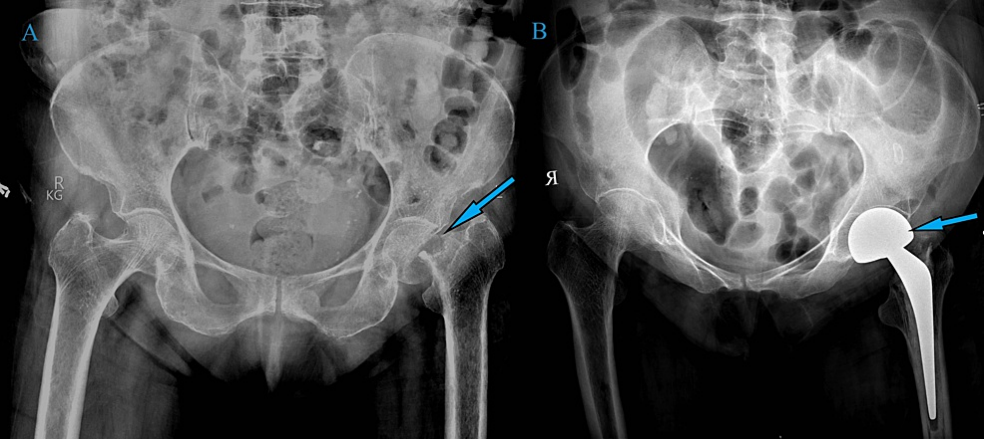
A) Preoperative pelvic X-ray showing displaced left femur neck fracture with significant osteoporosis B) Postoperative pelvic X-ray showing cemented left hip bipolar hemiarthroplasty at six-month follow-up
